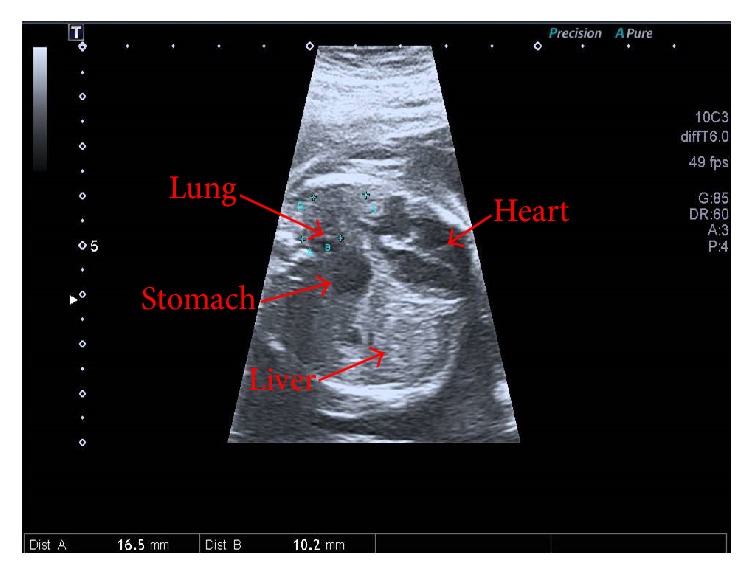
Ultrasound image. Ultrasound image in which we observe stomach and liver in the chest cavity next to four-chamber view of the heart and left lung's volume reduced.
